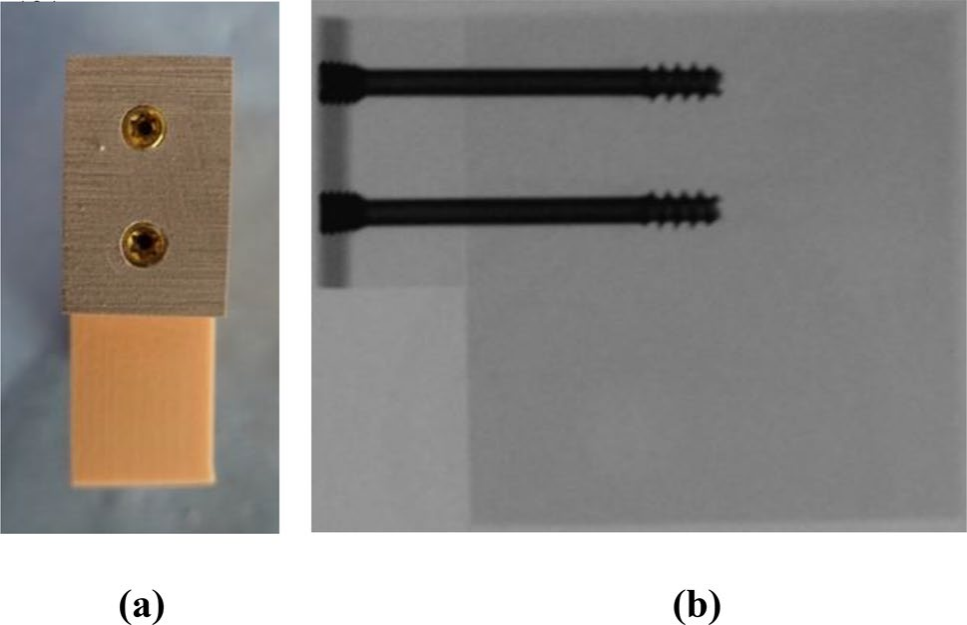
Prepared bone blocks with titanium screws before testing. (a) The frontal view; (b) X-Ray from the lateral view
